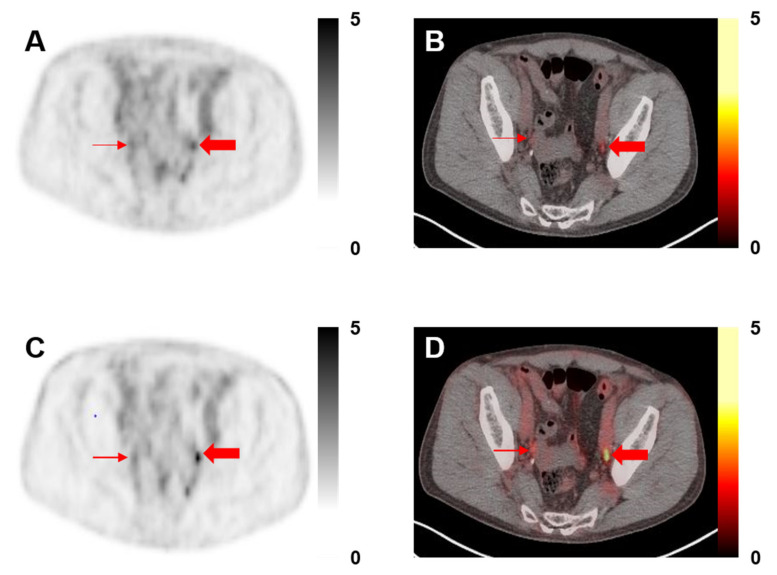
A 68-year-old man with history of prostatectomy and adjuvant chemohormonal therapy, now with an increase in prostate-specific antigen (PSA) to 0.3. (A) ^18^F-DCFPyL positron emission tomography (PET) and (B) PET/computed tomography (CT) axial images without point-spread function (PSF) demonstrating bilateral PSMA-RADS-3A pelvic lymph nodes (thin and thick red arrows, maximum standardized uptake value (SUV_max_) 1.2 on the right and 1.5 on the left). (C) ^18^F-DCFPyL PET and (D) PET/CT axial images with PSF show that the right pelvic PSMA-RADS-3A lymph node does not appear significantly more conspicuous (SUV_max_ 2.2) and that lesion was not re-categorized. However, the lymph node on the left (SUV_max_ 2.5) was thought to be more conspicuous and was re-categorized to PSMA-RADS-4.
